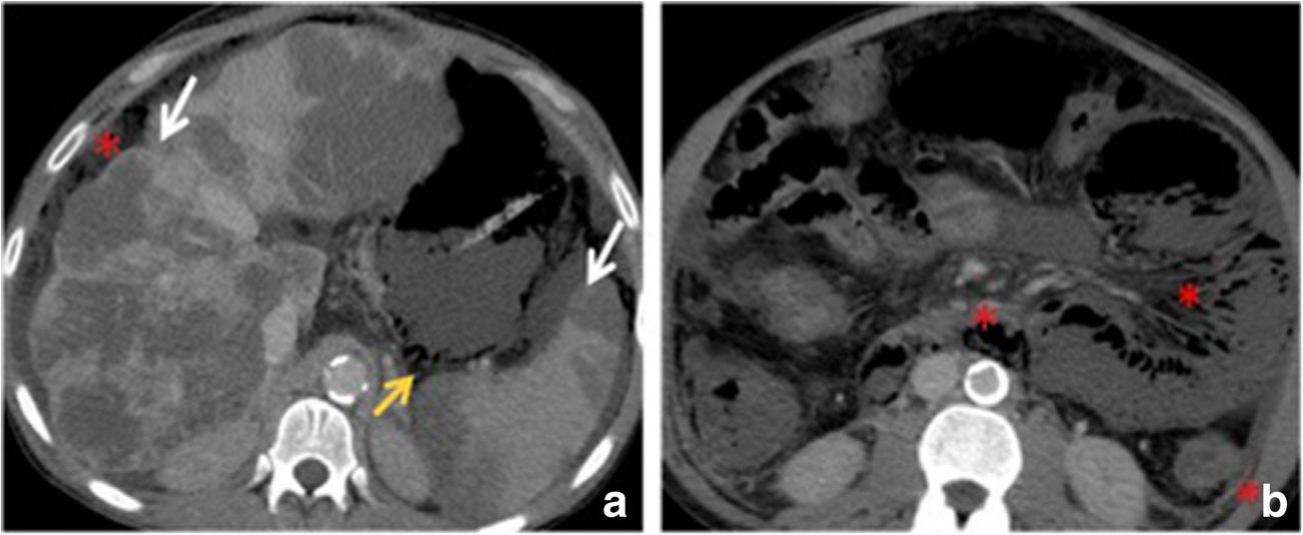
A 56-year-old male with mesenteric infarction. Axial enhanced CT shows various hypointense rounded regions of infarction in the hepatic parenchyma and wedge shaped in peripheral spleen parenchyma (white arrows) in a patient who has a mesenteric infarction. It also shows pneumatosis involving the gastric wall (orange arrow) and diffuse pneumo- and retropneumoperitoneum (*)
